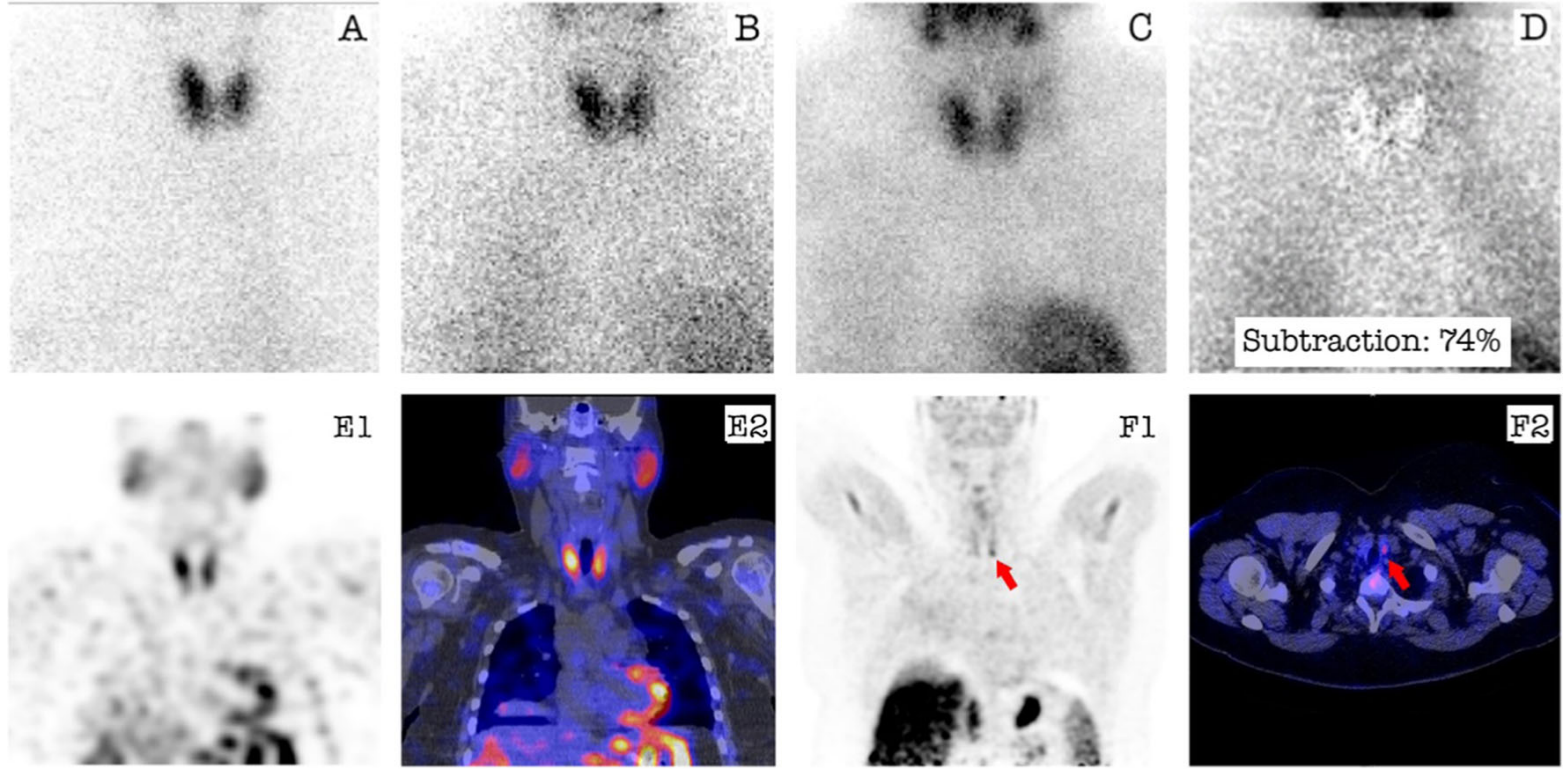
Patient example of a negative MIBI-SPECT/CT and a positive ^11^C-MET PET/CT. Planar anterior image of the neck with ^99m^Tc-pertechnetate (a), early ^99m^Tc-MIBI (b), and late ^99m^Tc-MIBI (c). Both planar subtraction image (early ^99m^Tc-MIBI minus ^99m^Tc-pertechnetate image (d)) and ^99m^Tc-MIBI SPECT-CT (E1-SPECT image only and E2 fused SPECT/CT image) do not show a clear focus suspect for adenoma. The ^11^C-MET PET/CT showed a small lesion located caudally from the left thyroid gland, suspicious for parathyroid adenoma (*red arrow* F1-PET image only and F2-fused PET/CT image)
